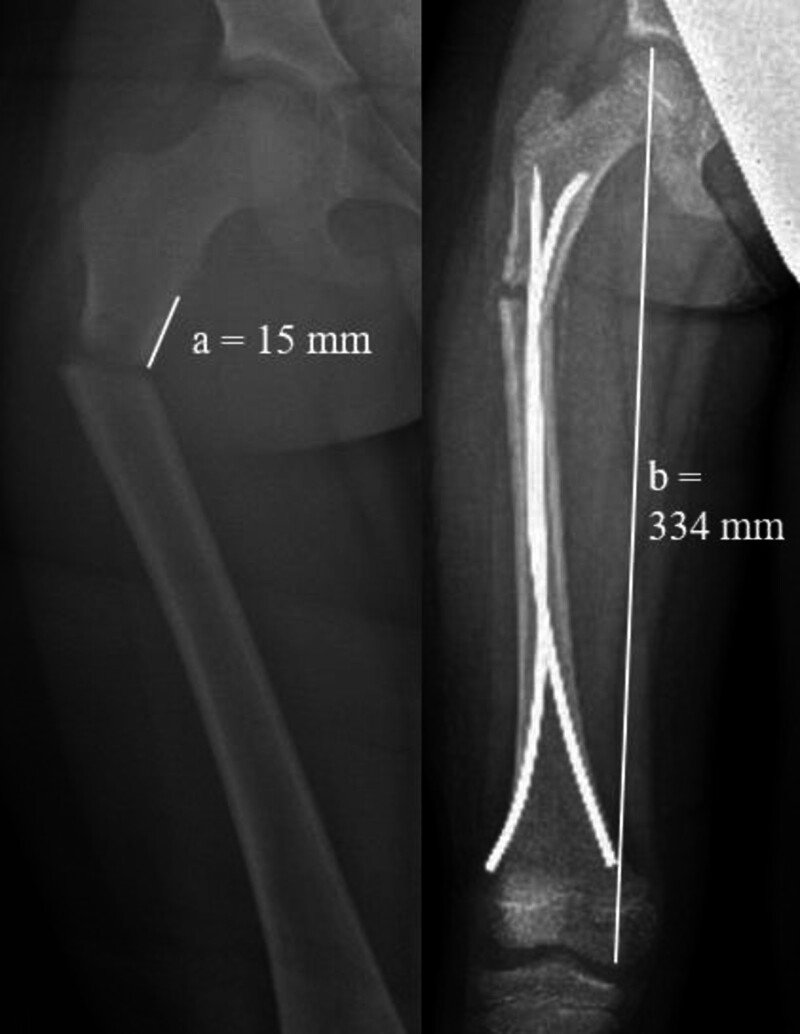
Radiographs demonstrating the method used to define short-segment subtrochanteric femur fracture, with (A) representing the distance between the inferior aspect of the less trochanter and the fracture site and (B) representing the total femur length, defined as the distance between the top of the femoral head and the medial femoral condyle. If (a/b) × 100 = <5%, the fracture was classified as short-segment. In this case, a = 15 mm, and b = 334 mm; 15/334 × 100 = 4%.
